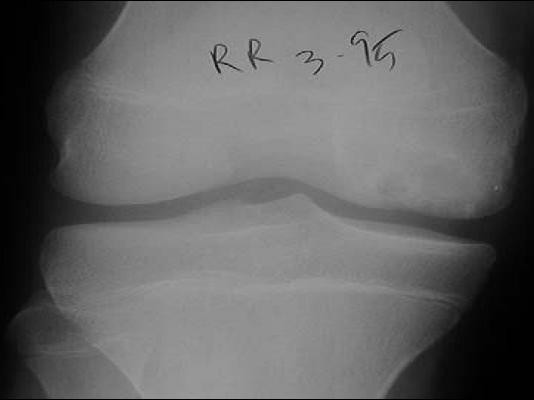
Osteochondritis dissecans. Anterior posterior  radiograph shows minimal cystic changes affecting the lateral aspect of  the medial femoral condyle [21].
